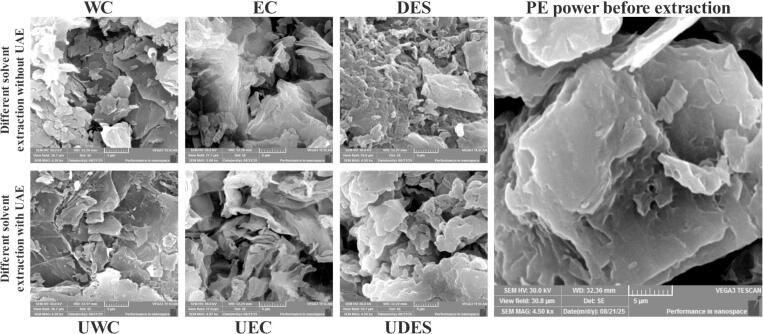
Microstructure of *P. eryngii* powder before extraction (PE powder) and after extraction using different solvents, with or without UAE examined at 4.5kx magnification with scale bars of 5 µm. Upper row: extraction without UAE using WC, EC, and DES. Lower row: extraction with UAE using UWC, UEC, UDES. Note: UAE (ultrasonic-assisted extraction); WC (water control); EC (ethanol control); UWC (water control with UAE); UEC (ethanol control with UAE); and PEPs (*Pleurotus eryngii* polyphenols).
